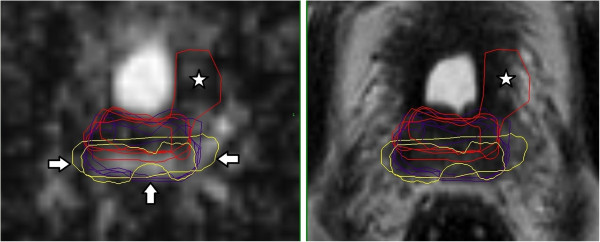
(Patient No. 5). Transversal slices through near the prostate base; left = DWI-, right = T2w-sequence. DWI based GTV-delineation (red contours) does not correspond to T2w based GTV-delineation (violet contours). Asterisk marks the erroneously contoured area in both pictures. Additionally erroneous delineation of unremarkable seminal vesicles (arrows in the left picture).
